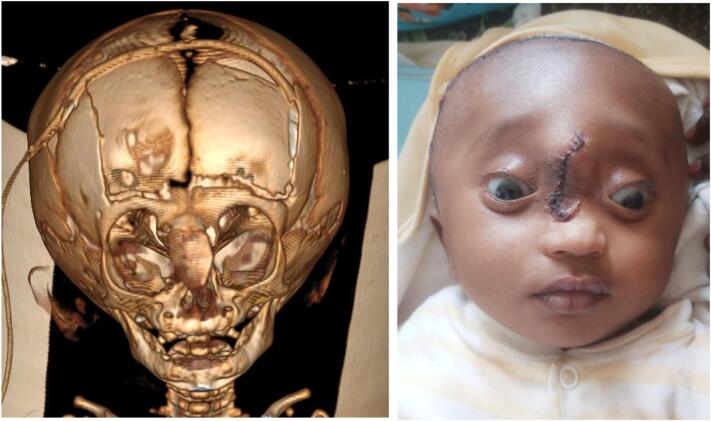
Postoperative follow-up CT scan and picture demonstrating good bone reconstruction and better aesthetic outcome.
